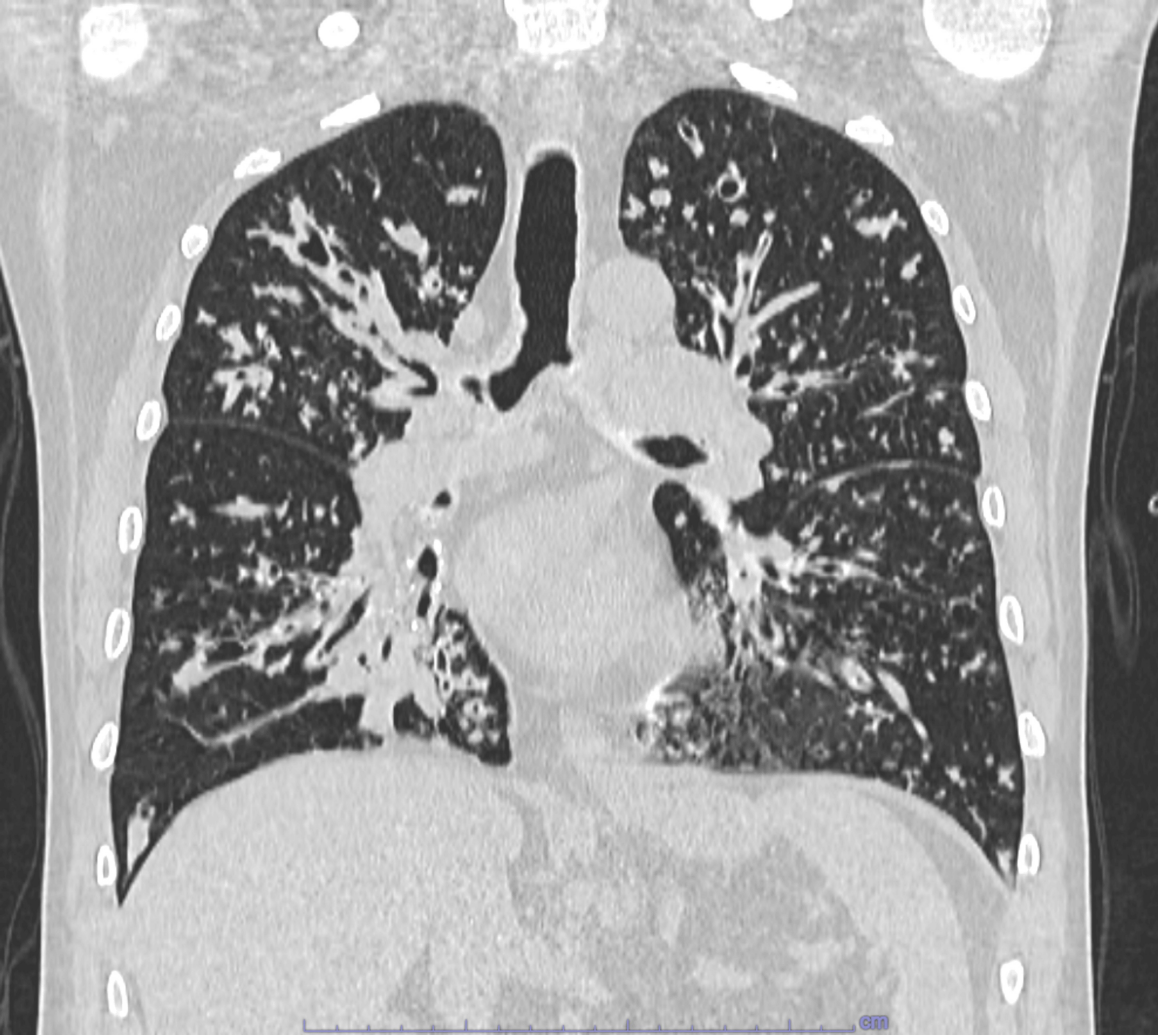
Chest CT scan performed in 2024 The CT scan shows progression of cylindrical and cystic bronchiectasis with predominant involvement of the upper lobes. Mucous infarcts of the bronchi and multiple peribronchial consolidations are also visible.
